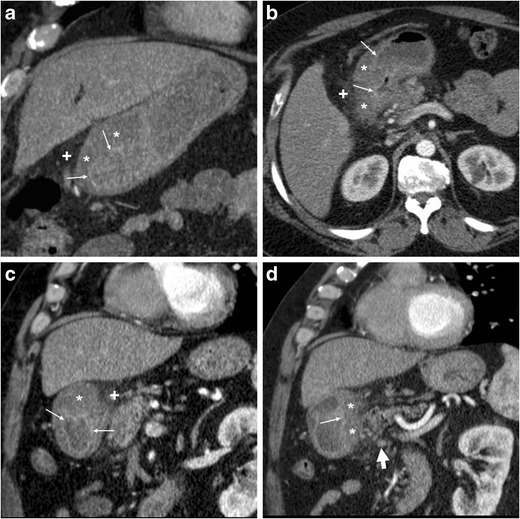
A 54-year-old obese male smoker, admitted to emergency department (ED) because of epigastric pain radiating to the back with deep abdominal tenderness, underwent urgent multidetector CT-angiography “to rule out acute aortic disease”. Secondary CT interpretation with multiplanar reconstructions identified findings consistent with peptic gastro-duodenitis, namely asymmetric oedematous mural thickening (*) with mucosal hyperenhancement (thin arrows) along the posterior and superior aspects of the distal antrum, pylorus and duodenal bulb, subtle perivisceral inflammatory stranding (+) and at least a centimetric lymphadenopathy (thick arrow in d) [Adapted from Open Access ref. no [10]]
